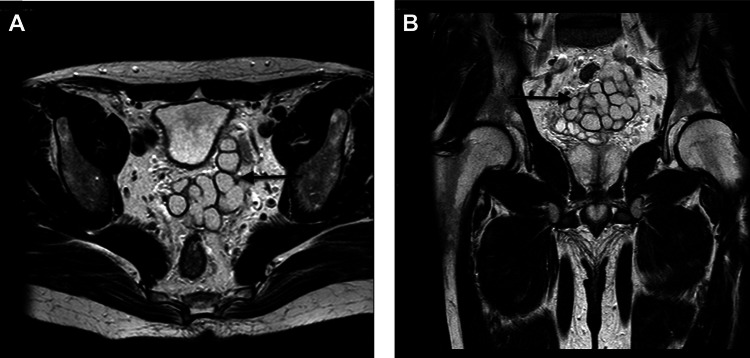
(A, B) MRI abdomen/pelvis revealed multiple cystic hyperintense structures in the region of the right seminal vesicle near the bladder base.
